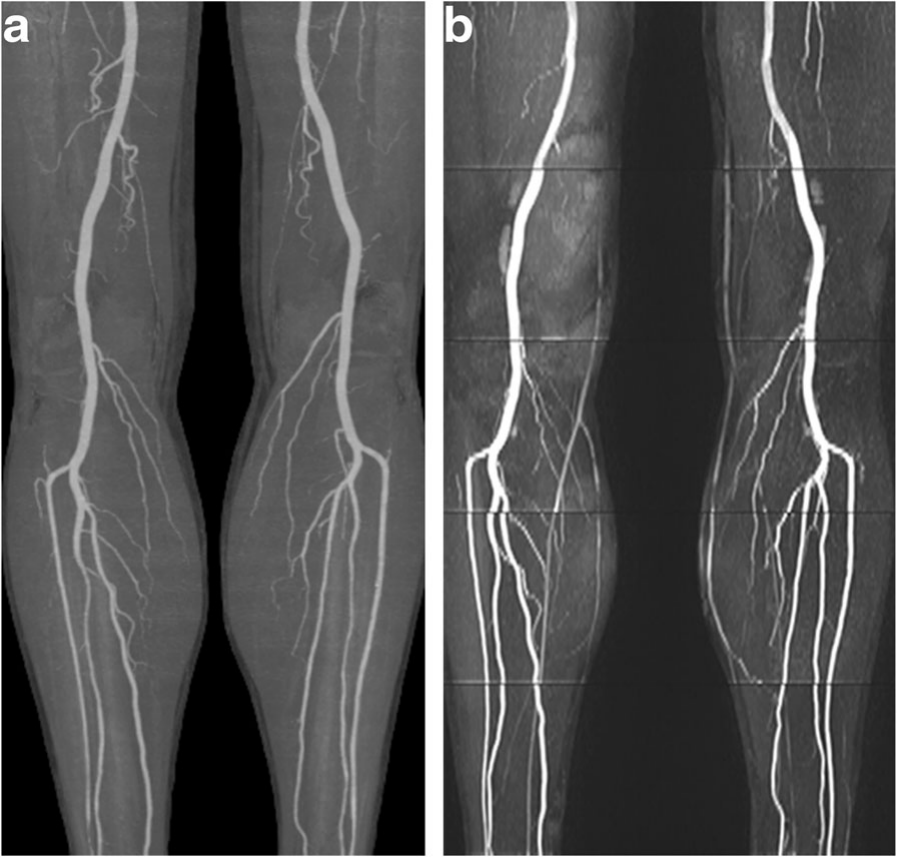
a Segments including popliteal artery, anterior tibial artery, posterior tibial artery and peroneal artery were rated as excellent in quality with CTA. b These segments were rated as excellent in quality with QISS MRA
